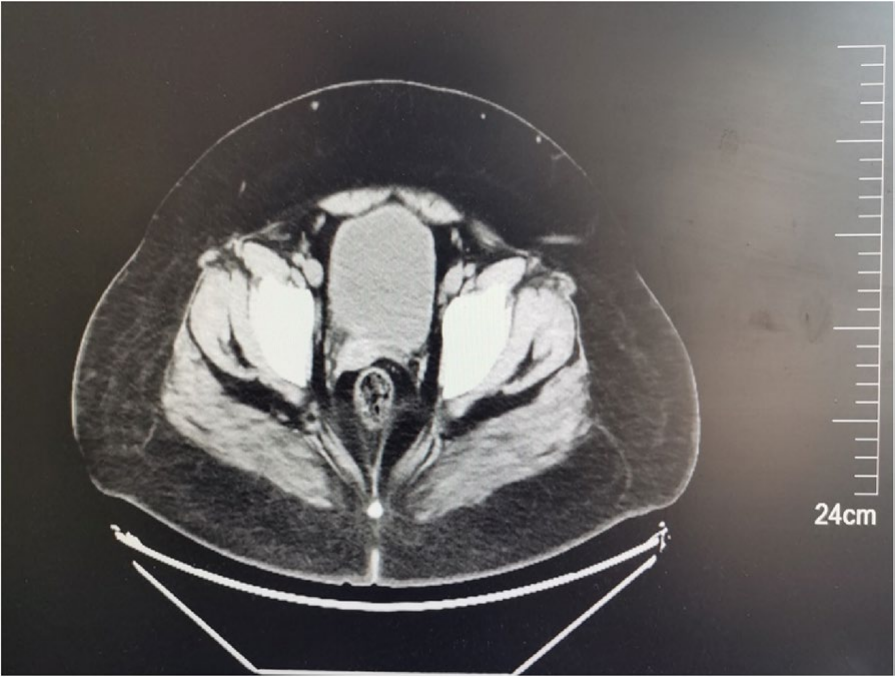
CT-scan (follow-up): The ovarian cyst disappeared
